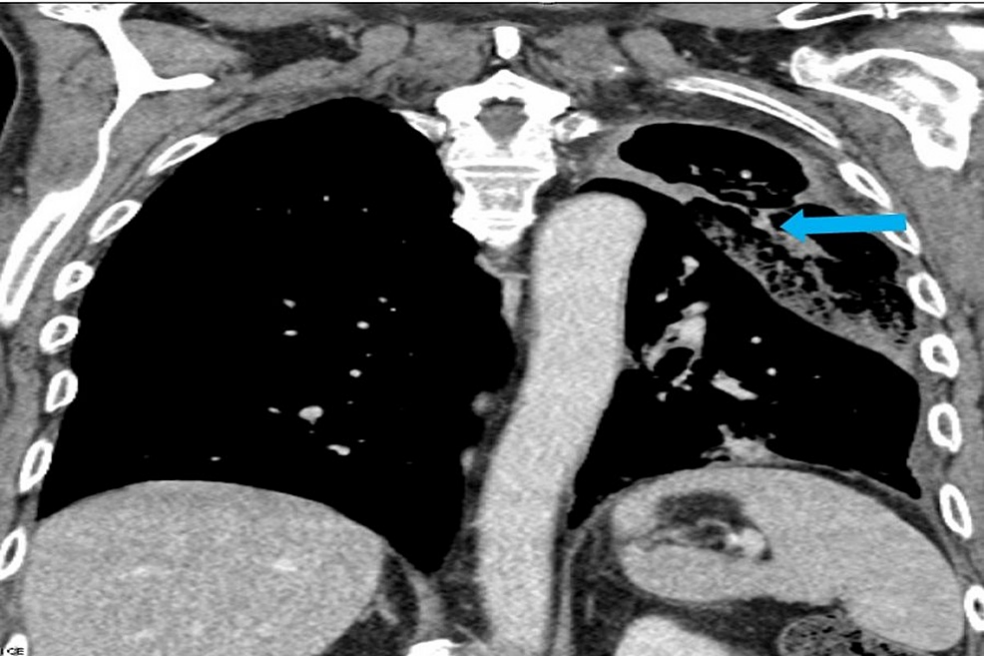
Computed tomogram (CT) Chest- Coronal view. Marked volume loss in the left upper lobe with a large cavity containing some soft tissue density material the appearances of which are those of an aspergilloma (blue arrow).
